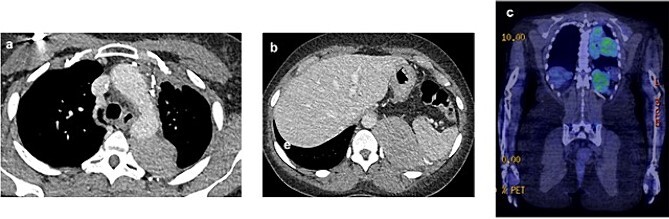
2021 PET and CT scan showing recurrence extension, involving the left parietal pleura (a, c) and the retroperitoneal tissue with spleen capsule (b).
